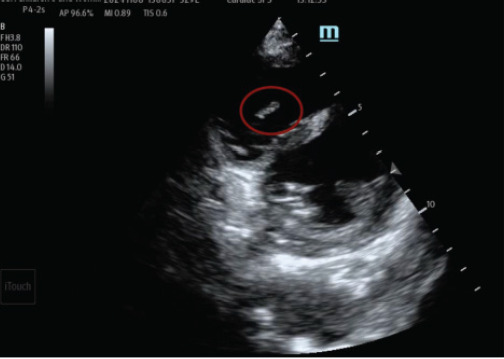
Hyperechoic structure (circled) on the tricuspid valve seen in parasternal short view of a point-of-care ultrasound.
